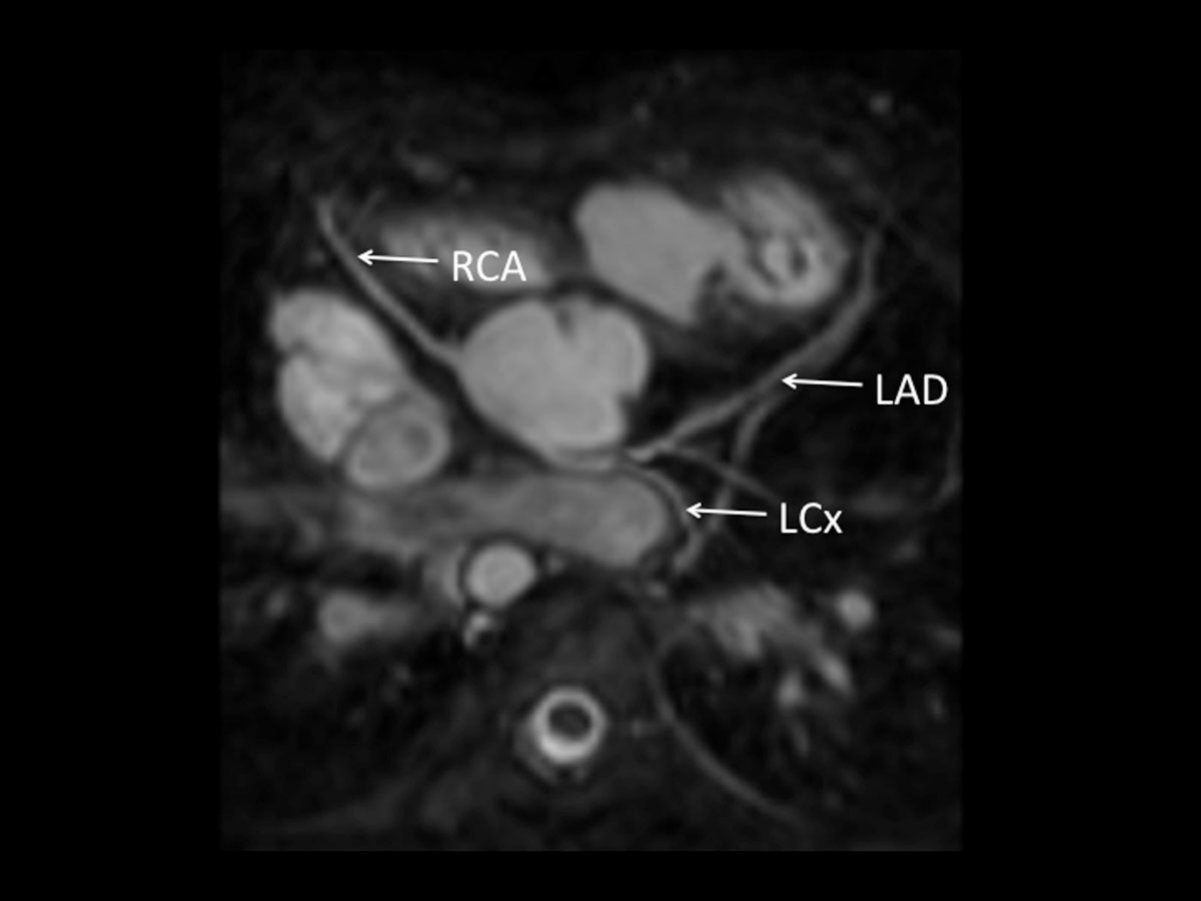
7 year old girl with repaired pulmonary atresia and ventricular septal defect. RCA arises from posterior non-coronary cusp. Also seen on echocardiogram
